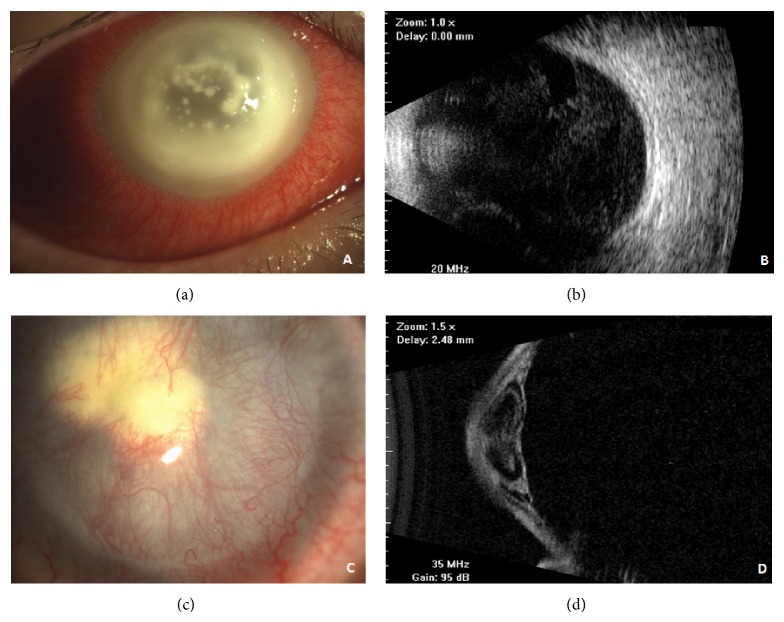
(a) Five days after CXL, slit-lamp examination revealed the presence of an annular corneal abscess, spared and scattered miliary infiltrates, and diffuse corneal edema with a strong inflammatory reaction in the ciliary body. The anterior chamber was not appreciable in detail. (b) Ultrasound B-scan showing vitreous reaction on postoperative day 7. (c) Slit-lamp image showing vascularized corneal pannus, with the presence of a dense leucoma in the center of the cornea. (d) Ultrastructural B-scan (UBM) evaluation showing the anteriorization of iris and lens planes, with the presence of angular synechiae and the absence of anterior chamber (2nd month after CXL).
